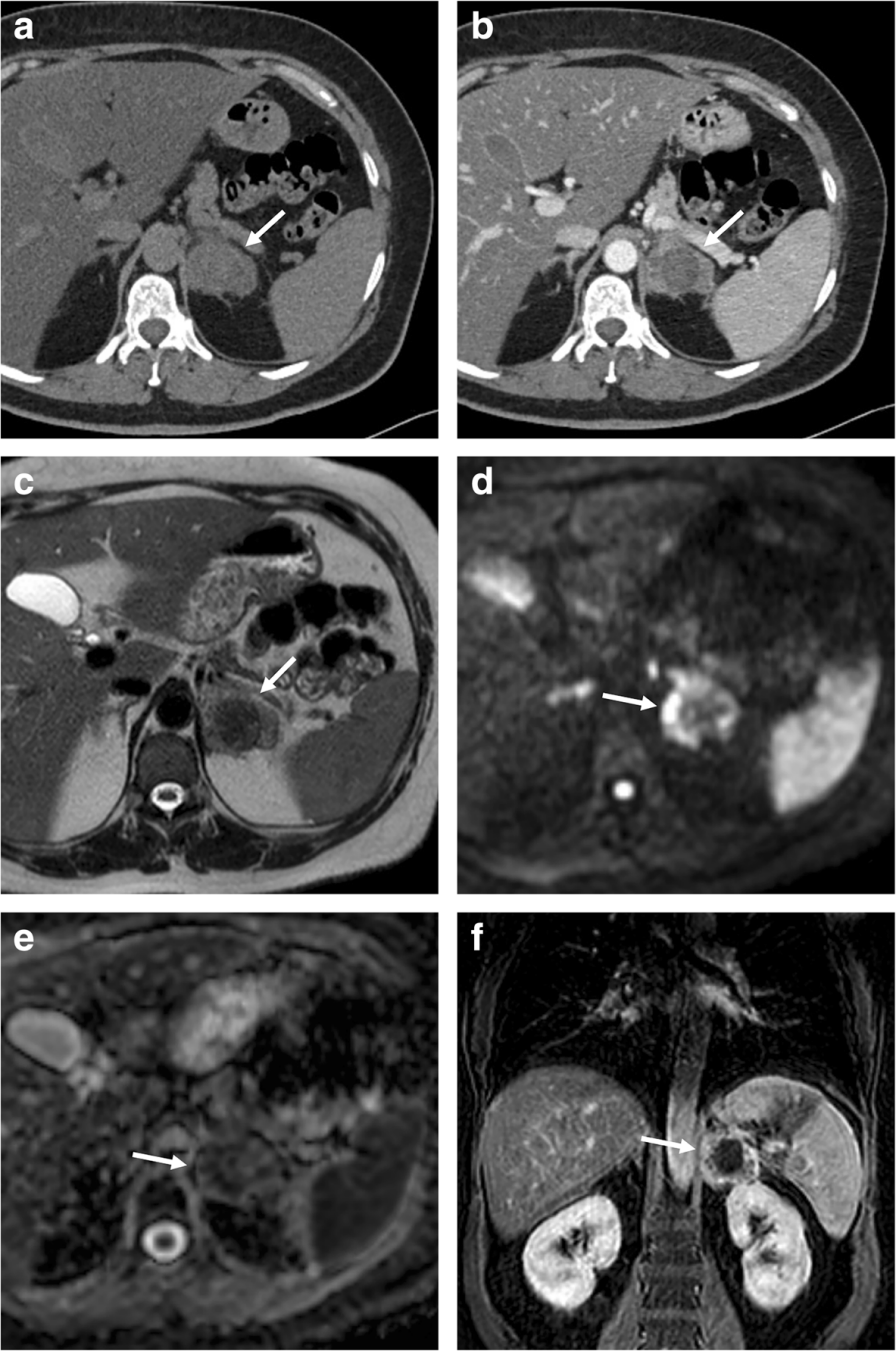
Left adrenal metastasis in a 58-year-old woman with breast cancer. Axial unenhanced CT (a), portal phase (b), T2-weighted fast spin echo (c), b800 s/mm^2^ diffusion-weighted image (d), ADC map (e), and coronal three-dimensional fat-suppressed T1-weighted gradient recalled echo image in portal phase after contrast injection (f) show a left adrenal mass (arrow). This lesion intra-lesional hemorrhage presenting as spontaneously hyperdense area on unenhanced CT (a), hypointense on T2-weighted (c), and no enhancement on CT (b) and MR (f) images after contrast injection. The peripheral solid component of the lesion shows also restricted pattern of diffusion (d, e)
